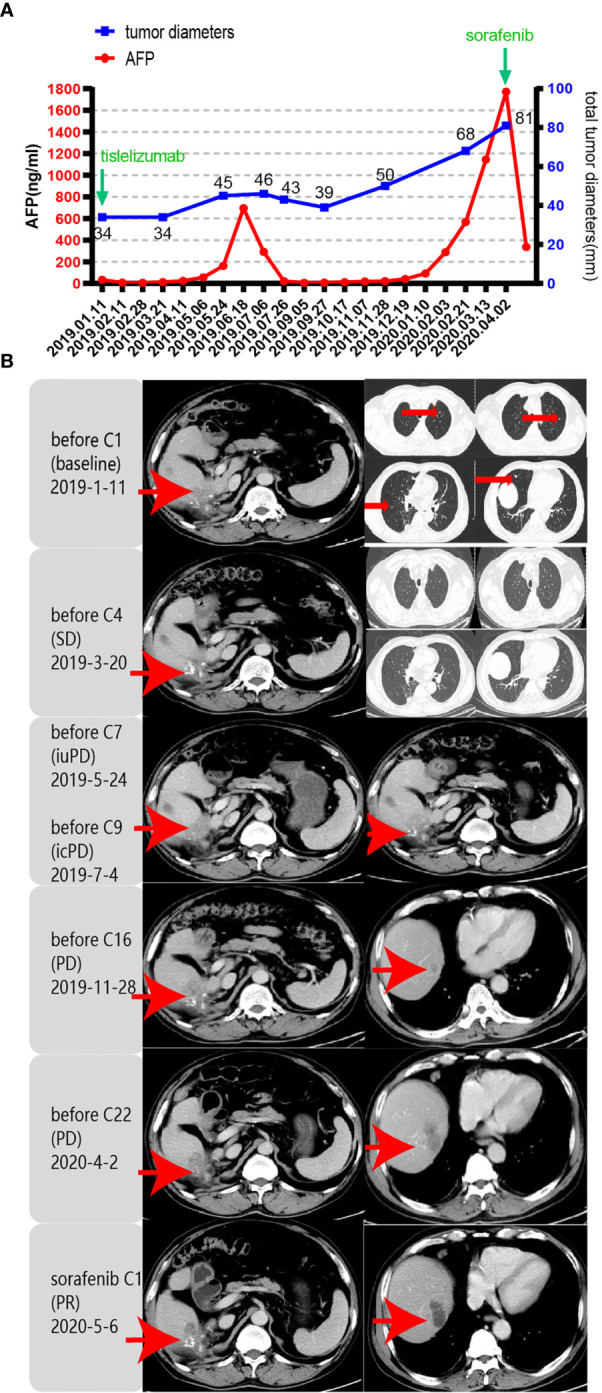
Response evaluation during the clinical course including changes in imaging and quantitative data. iuPD (immunity unconfirmed progressive disease); icPD (immunity confirmed progressive disease). (A) Trends in the levels of tumor monitoring indicators, including AFP (left Y-axis) and tumor diameters (right Y-axis) corresponding to the treatment timeline. X-axis showing the date of the disease course. The frequency of imaging evaluations is less than that of AFP. (B) Representative images of the CT scan revealed the increasing and decreasing process of both primary and metastatic lesions in the liver and lung after PD-1 antibody (tislelizumab) and sorafenib treatment. Red arrows indicate tumor lesions.
